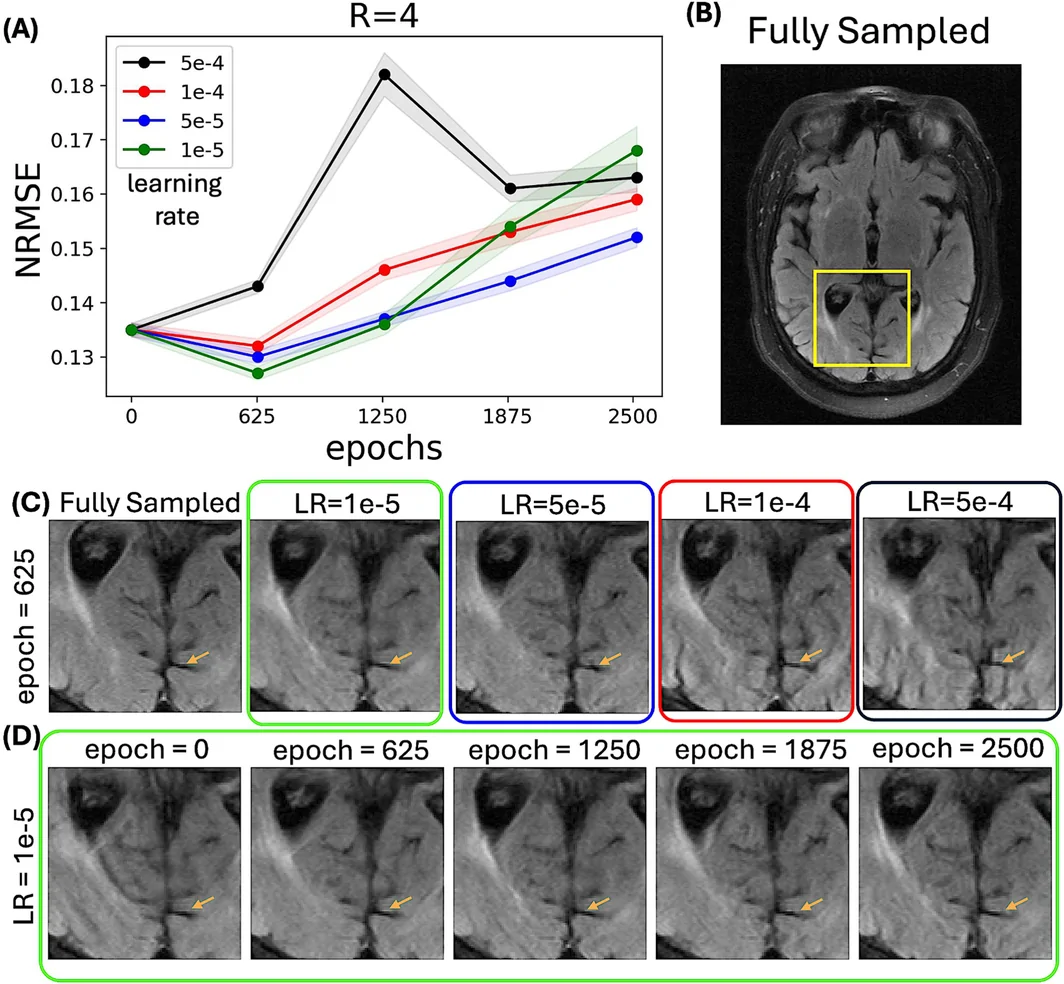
A representative example from the fastMRI FLAIR experiment. (A) Quantitative NRMSE as a function of fine‐tuning duration for different learning rates, highlighting that a comparatively smaller learning rate and fewer fine‐tuning epochs yields the best performance. (B) Fully sampled reference FLAIR image. (C) Zoomed reconstructions obtained using different learning rates with 650 fine‐tuning epochs, compared against the fully sampled reference. (D) Reconstructions obtained using a fixed learning rate of $1\times 10^{-5}$ across different fine‐tuning durations. Appropriate fine‐tuning hyperparameters lead to visibly improved structural fidelity and reduced artifacts, as highlighted by the orange arrows.
